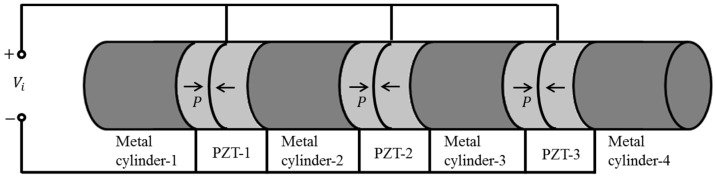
The cascaded piezoelectric ultrasonic transducer with three sets of piezoelectric ceramic stacks.
